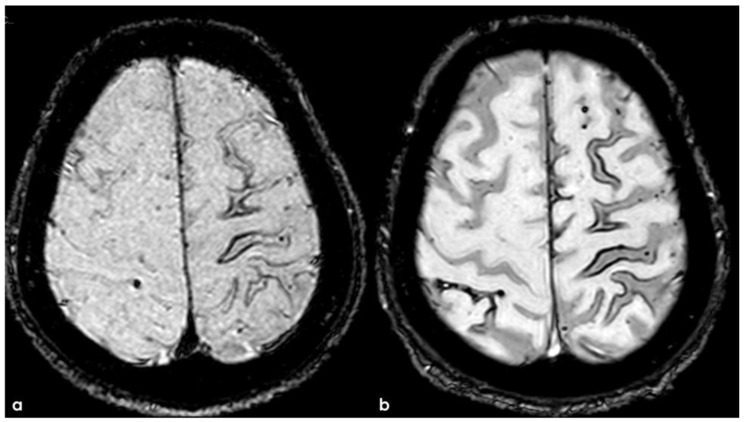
SWI MRI in 2015 (panel (a)) and in December 2017 (panel (b)), showing the evolution of sulcal involvement in the right post-central sulcus.
